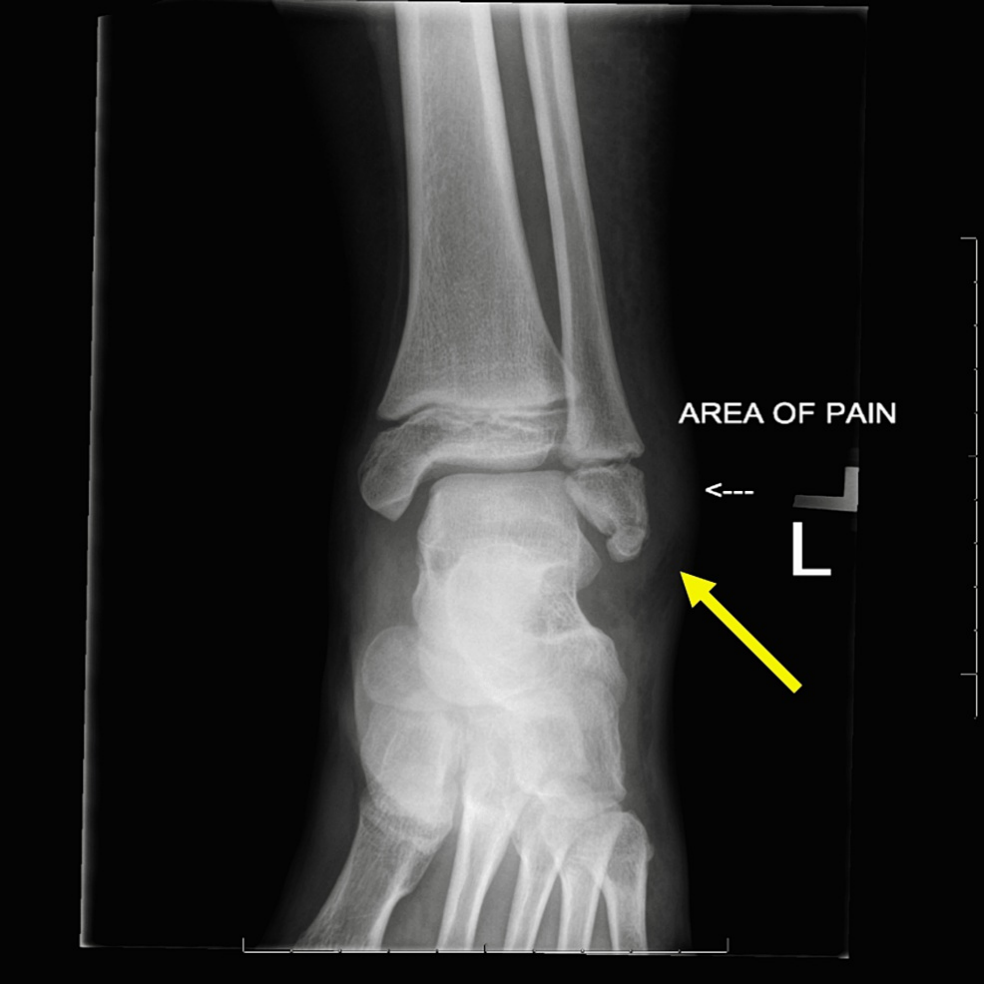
Anterior-posterior (AP) radiograph of the left ankle shows a fracture line and a reported area of pain at the distal lateral malleolus (yellow arrow) two years prior to presentation.
